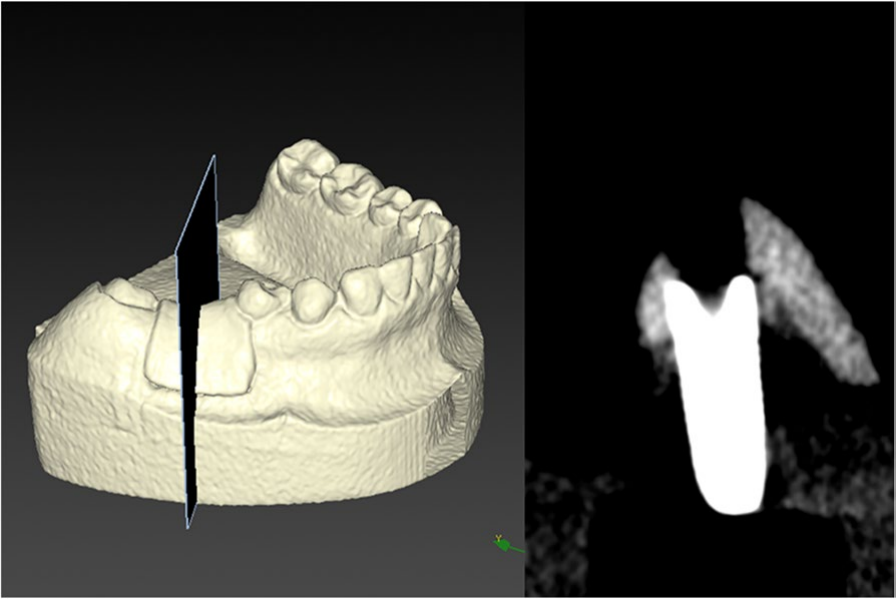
3D reconstruction of postsurgical CBCT (left; STL-CBCT) and its 2D cross-section of implant (right)
